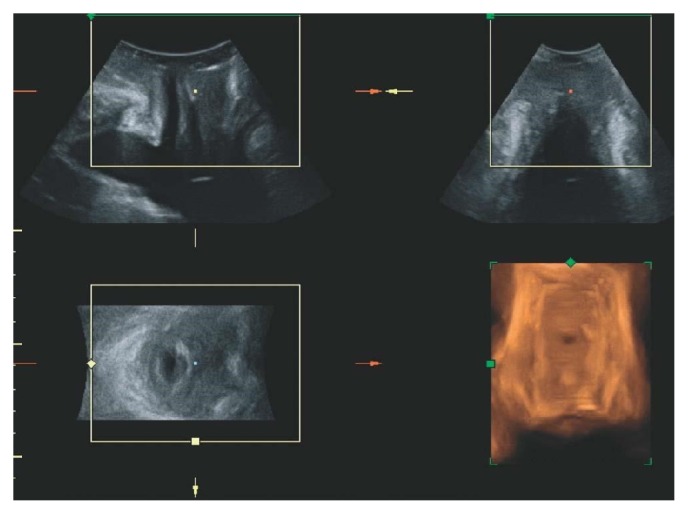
Lower urinary tract on three-dimensional transperineal ultrasound imaging in a woman with tension-free vaginal transobturator tape. The top left image shows the midsagittal view (A-plane), with the tape visible as a hyperechogenic stripe just ventral (left) of the central marker dot. The top right image is a coronal view (B-plane) and the bottom left is the axial or C-plane, showing the tape as a shallow “v” (rotated 90° anticlockwise) to the left of the central marker. The bottom right image is the resulting rendered volume [12].
